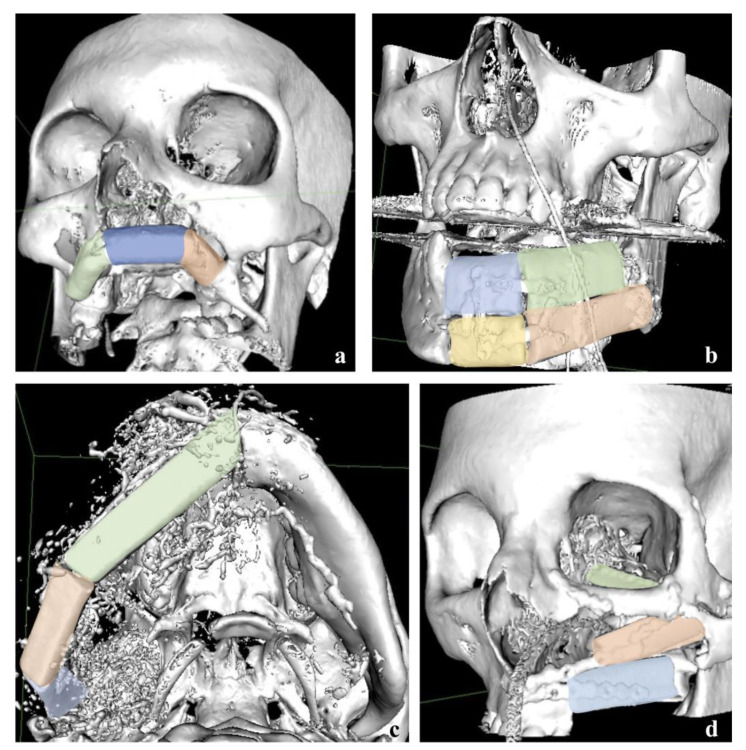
Examples of challenging reconstructive cases. (a) Three-pieces reconstruction of the whole maxilla due to excision of a chondrosarcoma in a young girl. (b) Double-barreled reconstruction of left body of the mandible following removal of an ameloblastoma. (c) Reconstruction of right hemimandible with free fibula flap in a three-pieces fashion. A widely extended arterio-venous malformation was removed after its embolization. Embolizing material artifacts are visible in the CT. (d) Midface reconstruction achieved by means of a free fibula flap in three segments after having removed a massive psammomatoid ossifying fibroma.
